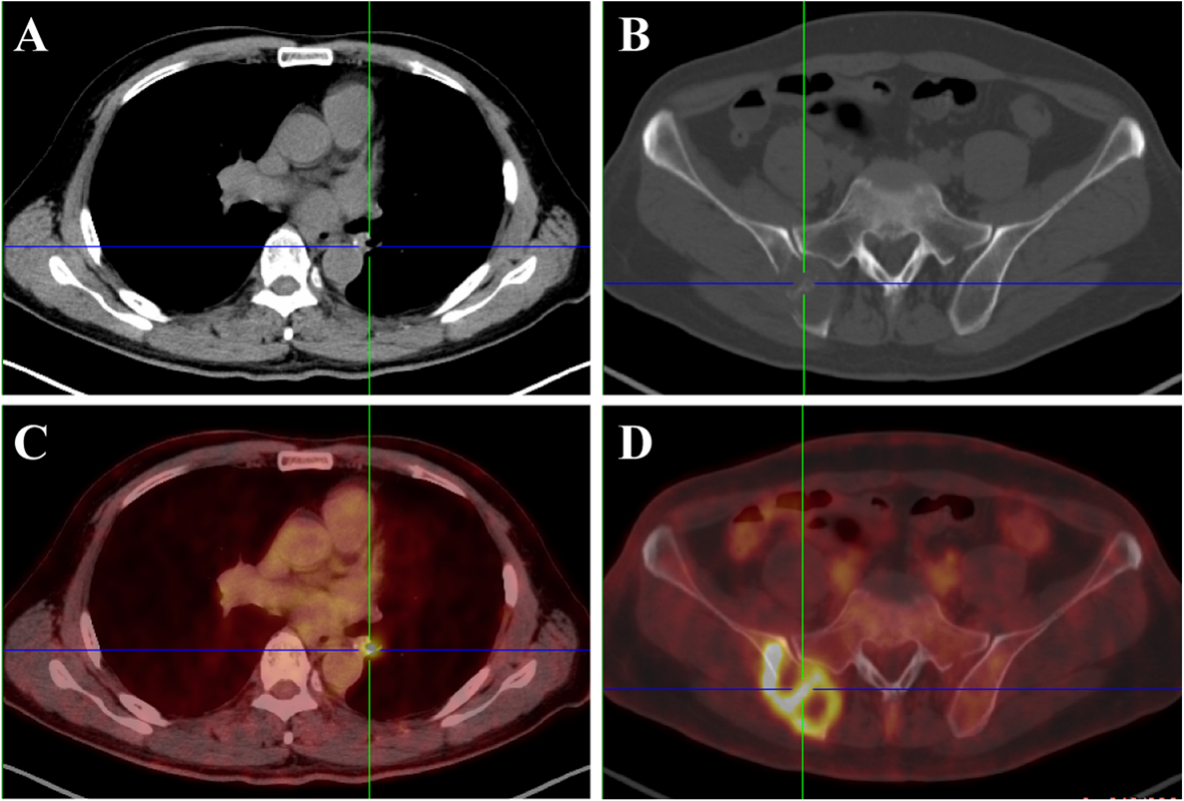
PET/CT scan showed typical imaging alternations in the anastomotic region (a, c) and right iliac bone region (b, d), which was suspected of disease recurrence
